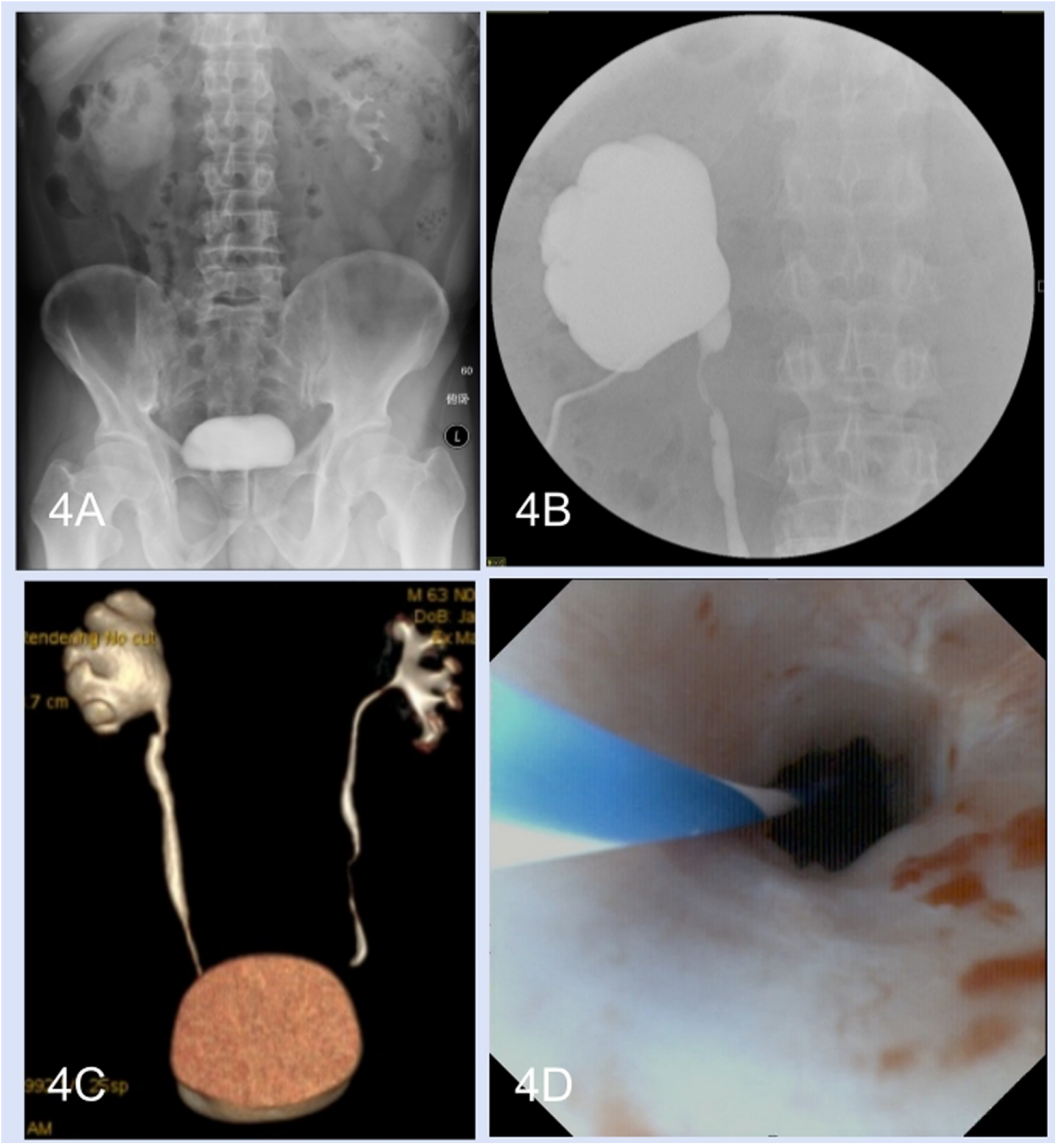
Perioperative radiological examinations and postoperative ureteroscopy. (A) Preoperative intravenous urography. (B) Anterograde pyelography. (C) Postoperative computed tomography urography. (D) Postoperative ureteroscopy.
